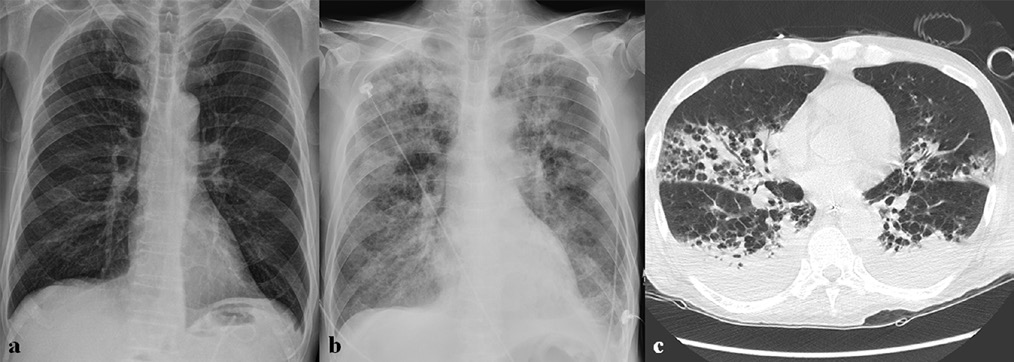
A 68-year-old male with MERS-CoV infection. (a) Initial chest radiograph (CXR) obtained 1 day after symptom onset shows ill-defined patchy ground-glass opacities (GGOs) in both middle to lower lung zones. (b) Follow-up CXR obtained 14 days after symptom onset shows rapid progression with bilateral diffuse GGOs and consolidation in both lungs. Also note blunting of left costophrenic angle, suggesting pleural effusion. (c) Follow-up chest CT obtained 18 days after symptom onset shows bilateral consolidation and GGOs with bilateral pleural effusion. He died of respiratory failure.
